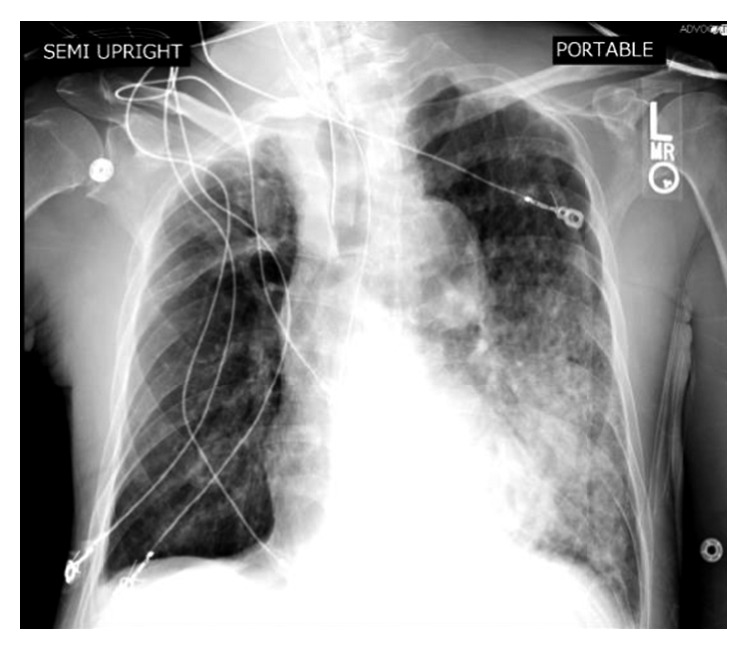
Chest X-ray showed patchy airspace infiltrates in the left lower lobe suggestive of pneumonia.
